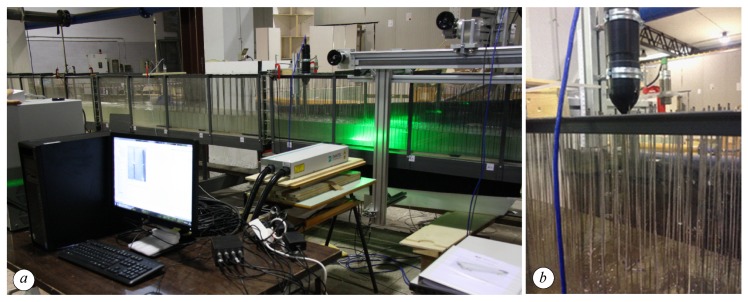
(a) View of the flume section with the Particle Image Velocimetry (PIV); (b) the particulars of an ultrasonic probe and ADV.
